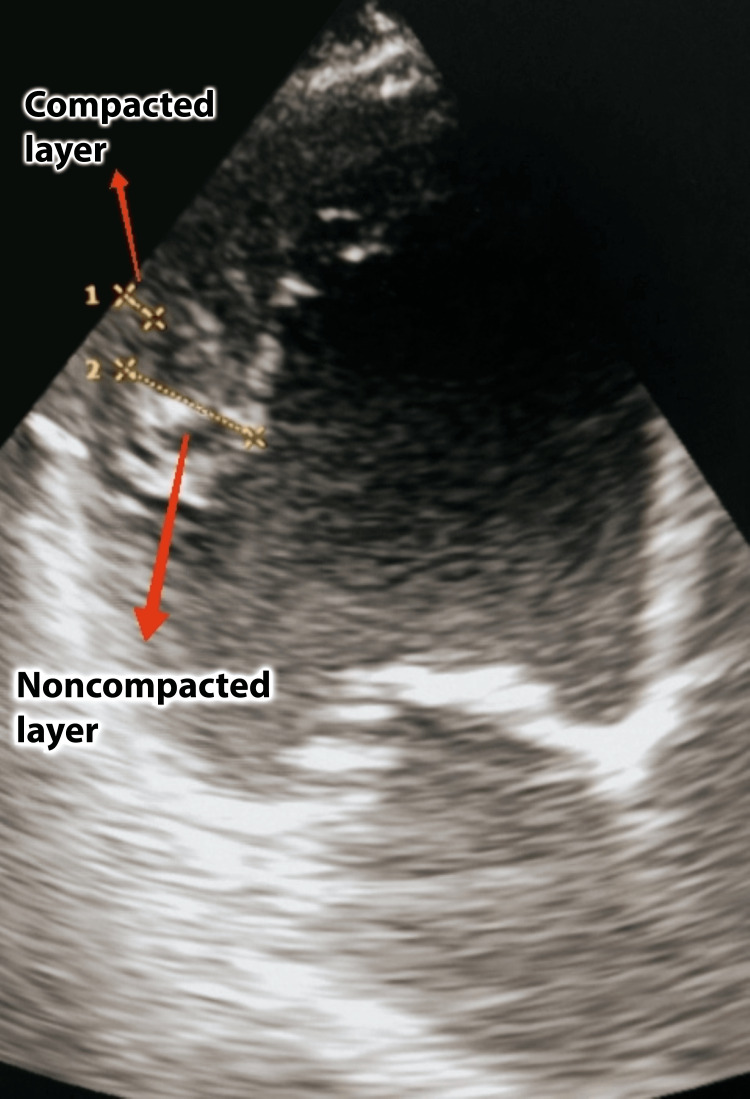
Echocardiography, apical four-chamber view. Multiple trabeculations in the middle and apical segments of the left ventricle. A NC/C in the midventricular segments >2.5:1. NC/C: noncompacted to compacted myocardium ratio. 1 = 0.7 cm; 2 = 1.8 cm.
